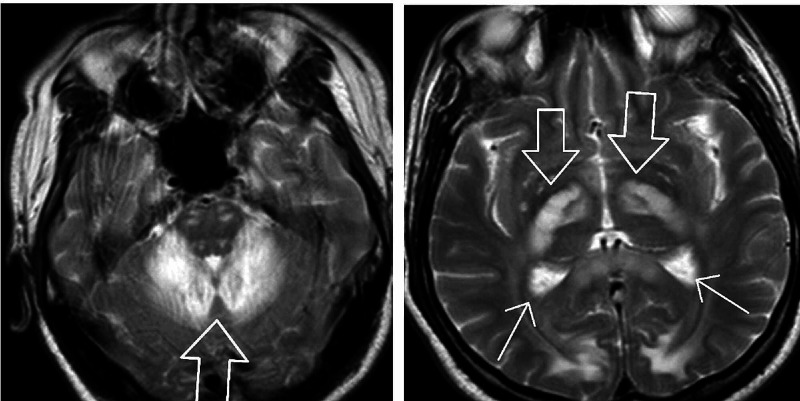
MRI brain without contrast
